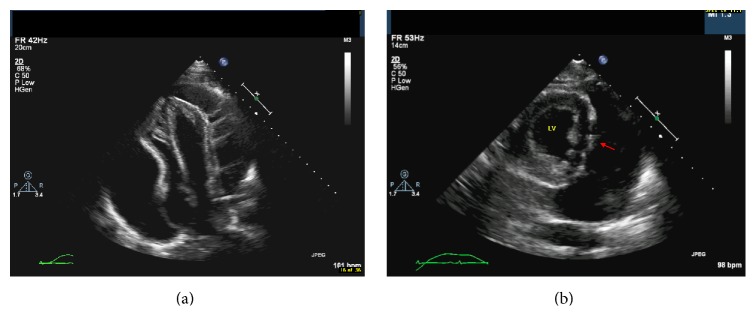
(a) Apical 4-chamber view on transthoracic echocardiogram showing large circumferential pericardial effusion with right atrial and ventricular compression. (b) Parasternal long-axis echocardiographic view of the left ventricle showing straightening of the LV lateral wall in diastole (red arrow).
